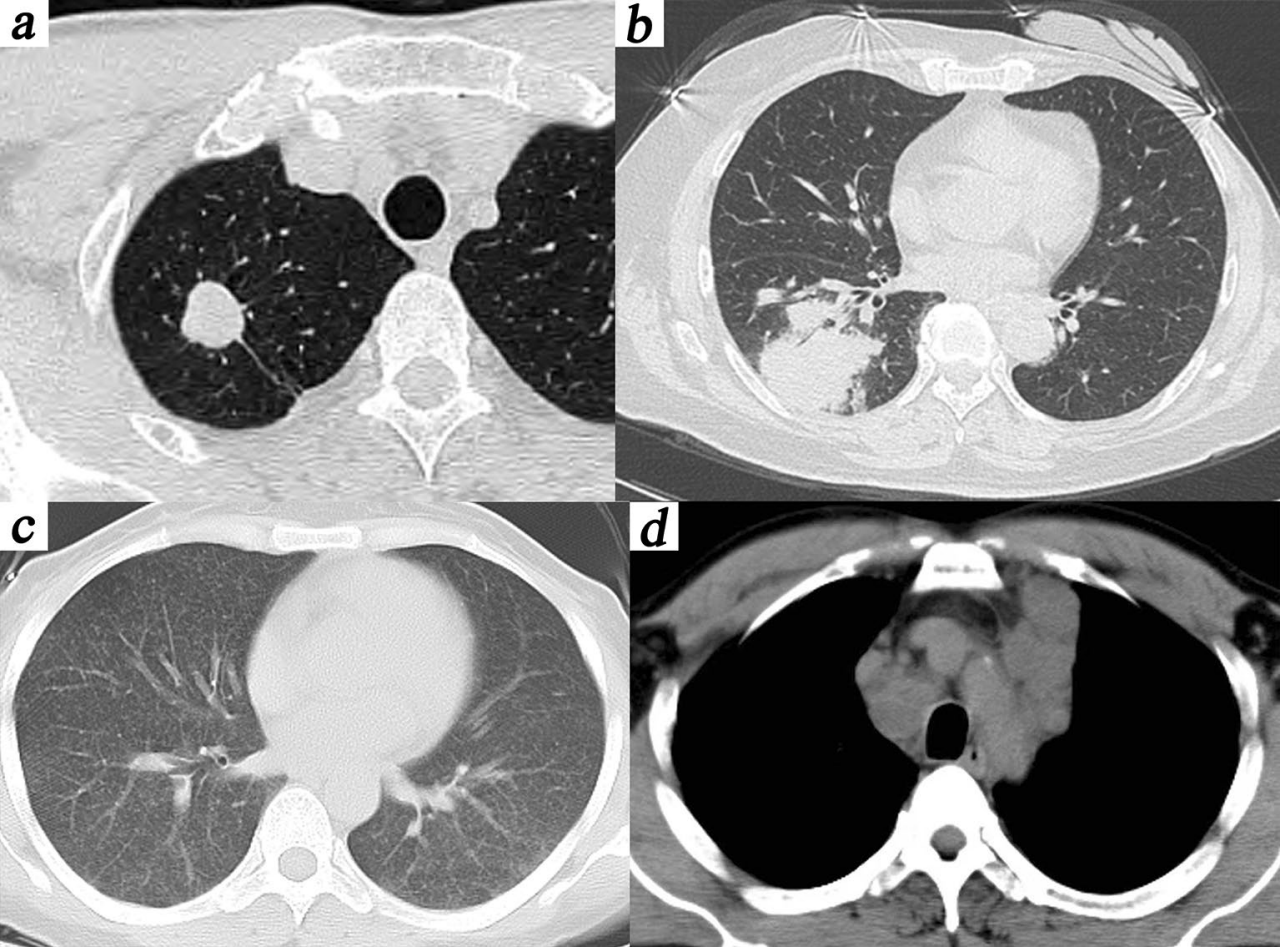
The CT manifestations of PC patients: (a) A 41-year-old male with normal immune function indexes was asymptomatic. Nodular type: A single nodule with smooth margins and uniform density was seen in the upper lobe of the right lung. (b) A 58-year-old female, after breast cancer surgery, was infected with Cryptococcus in the lungs during chemotherapy, with symptoms of fever and cough. Consolidation and air bronchial signs were seen in the right lower lobe. (c) A 38-year-old female presented with a pulmonary infection of Cryptococcus during corticosteroid therapy, with fever and cough. Miliary pattern: Multiple miliary nodules were seen in both lungs. (d) A 50-year-old male was immunocompromised and had cough. Mediastinal lymphadenopathy was seen in her lung CT.
